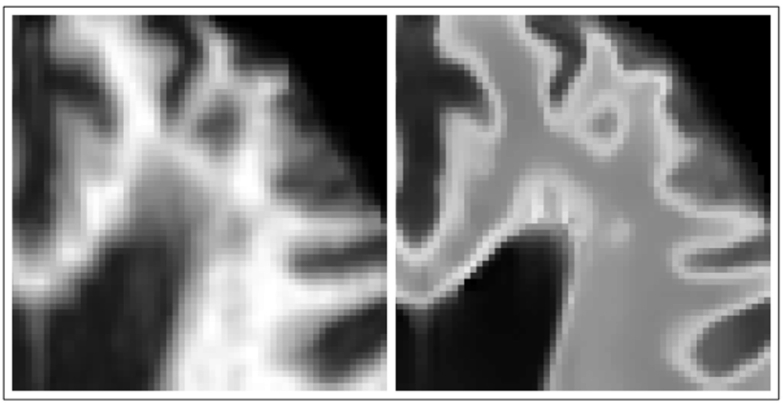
An example where periventricular WMH has been synthesised. Left: Normalised *T*_1_-w image. Right: Corresponding synthetic FLAIR image.
